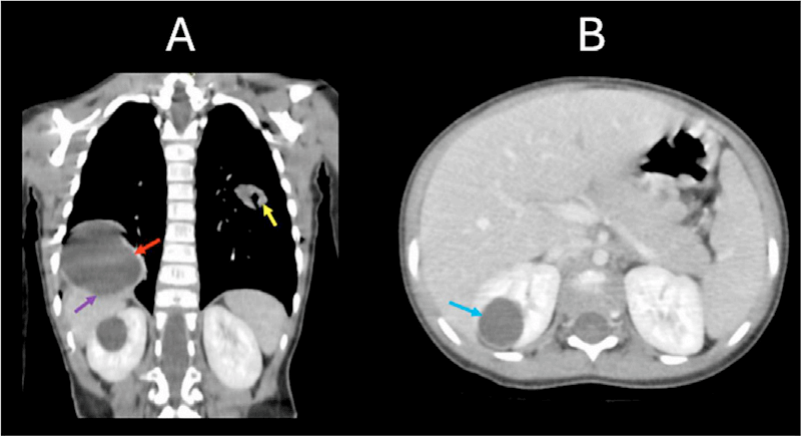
Contrast-enhanced CT scan in the portal venous phase. (A) Coronal reconstruction at the thoracic level demonstrates 2 cystic lesions: a well-defined, round, fluid-filled cyst in the right lung (red arrow), causing inferior displacement of the diaphragm and indentation of the hepatic contour (purple arrow); and an oval-shaped, cavitary lesion with internal air lucency in the left lung (yellow arrow), suggestive of partial cyst rupture. (A & B) Coronal (A) and axial (B) views also reveal a well-circumscribed, round cystic lesion in the upper pole of the right kidney (blue arrows), with homogenous fluid content and a thin, regular wall.
